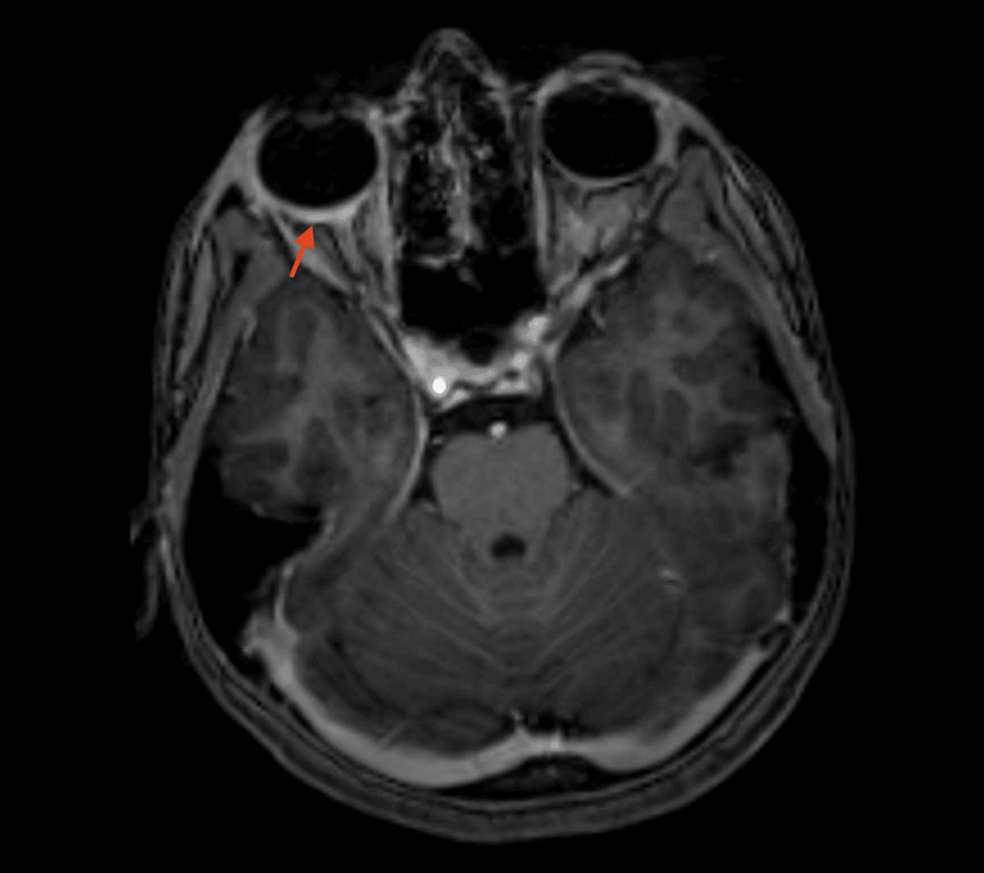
MRI of the brain and orbit (axial T1 post-contrast image). Thickening and enhancement of the posterior sclera of the right eye (red arrow).
